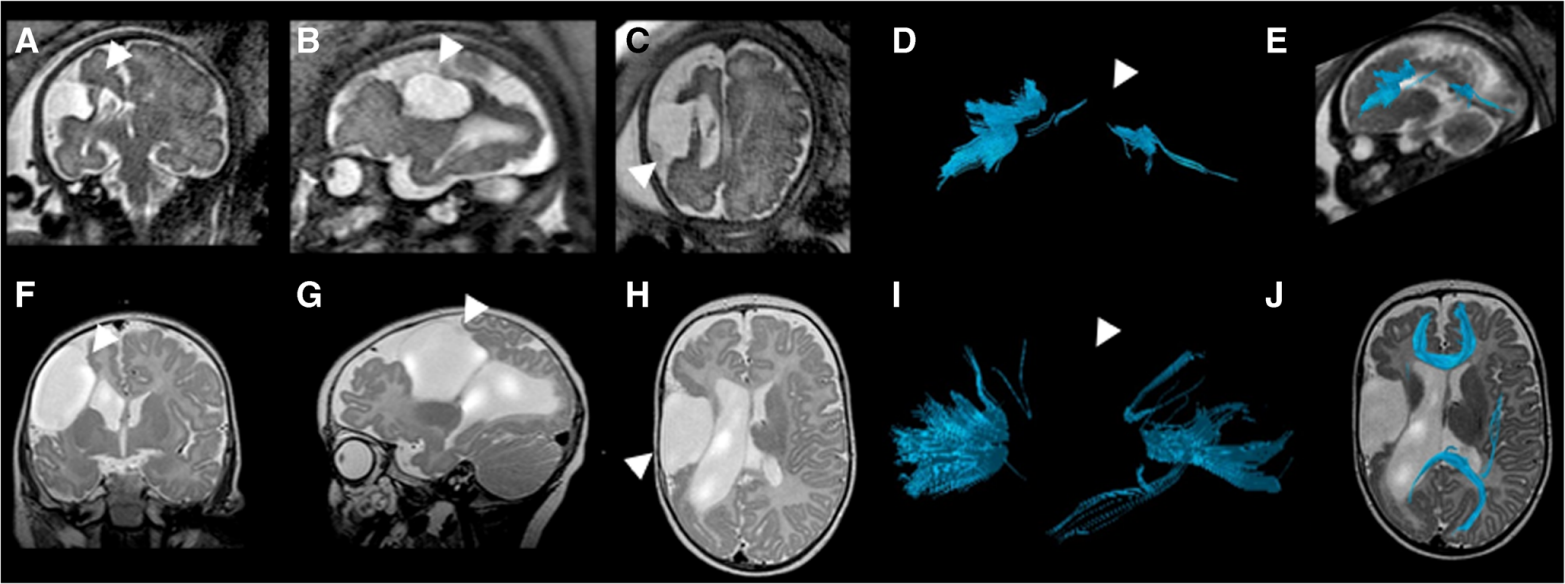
Fetal and postnatal corpus callosum segmentation in a boy (case 12) with right middle cerebral artery territory infarct. a–e Age 33+6 gestational weeks. a Coronal T2-weighted (TR/TE 2,042/140 ms, flip angle 90°) MR image of the brain reveals cystic encephalomalacia in the region of the right middle cerebral artery infarct (*arrowhead*). b Sagittal T2-weighted image of the fetal brain shows infarct (*arrowhead*). c Axial T2-weighted image of the fetal brain with infarct (*arrowhead*). d Sagittal view of the fiber tract segmentation reveals that the body of the corpus callosum is not visualized in the fetal diffusion tensor imaging (DTI; *arrowhead*). e Overlay of the callosal fiber tract on a sagittal T2-weighted image. f–j Age 4 months. f Coronal T2-weighted (TR/TE 3,000/120 ms, flip angle 90°) MR image of the brain shows sequelae of infarct (*arrowhead*). g Sagittal T2-weighted image shows infarct (*arrowhead*). h Axial T2-weighted image of the postnatal brain shows infarct (*arrowhead*). i Segmented sagittal DTI of the corpus callosum in the postnatal brain reveals that, similar to the fetal DTI, the body of the corpus callosum is not visualized. j An overlay of the segmented callosal fibers on an axial T2-weighted image reveals the markedly thinned parenchyma in the region of the right middle cerebral artery territory with cystic encephalomalacic changes. *TE* echo time, *TR* repetition time
